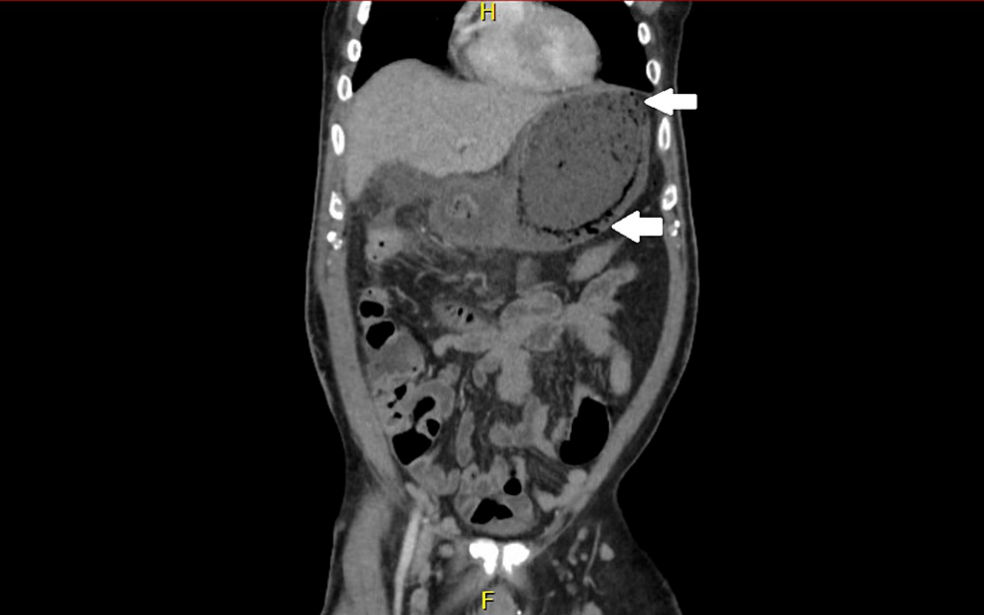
CT scan image of abdomen and pelvis demonstrating severe gastritis with pneumatosis and delayed emptying of the stomach
